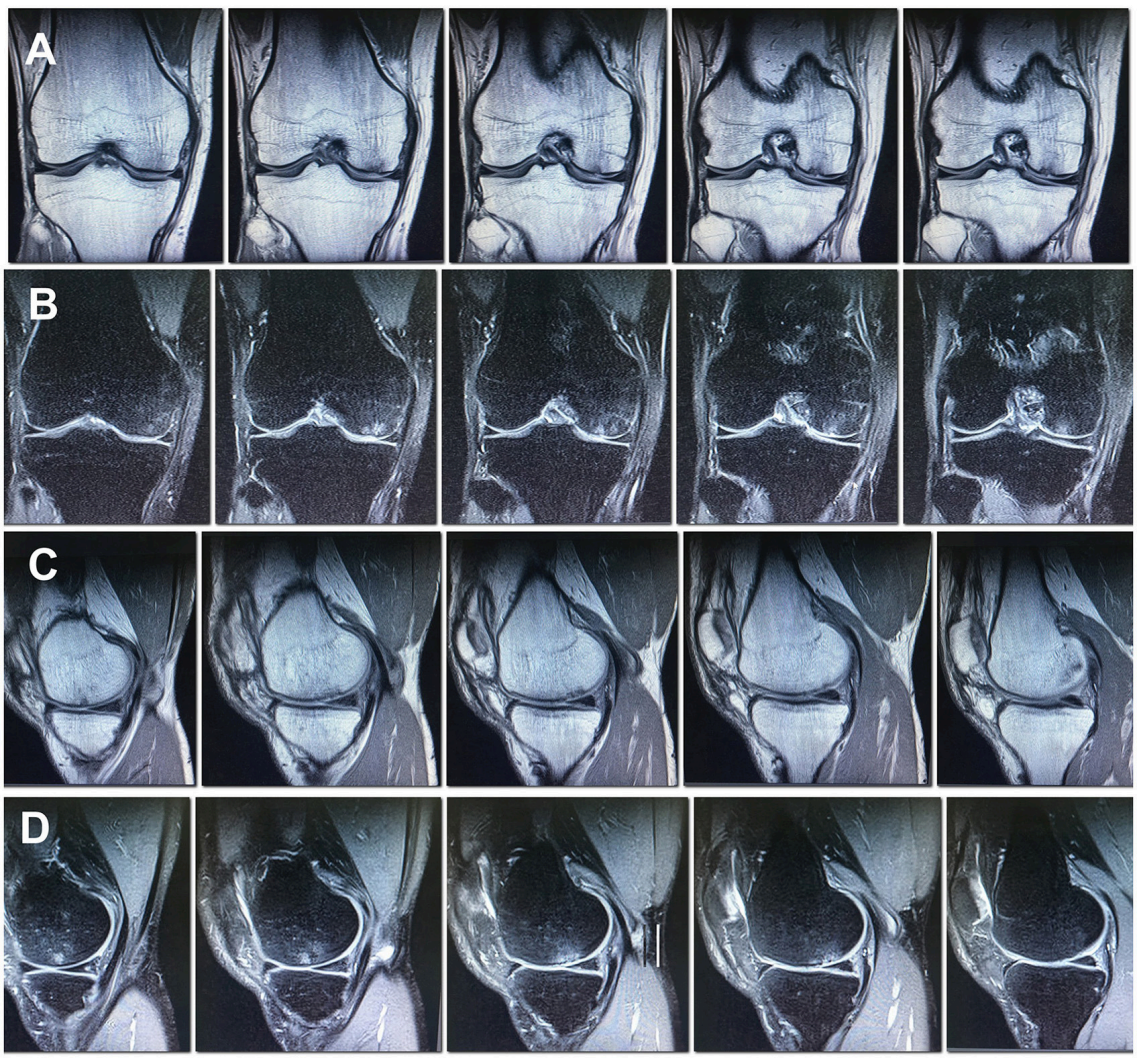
MRI of the right knee 10 months after the surgery. Decreased subchondral edema. (A) Cor T1FSE; (B) Cor PD fat sat FSE; (C) Sag T1FSE; (D) Sag PD fat sat FSE.
